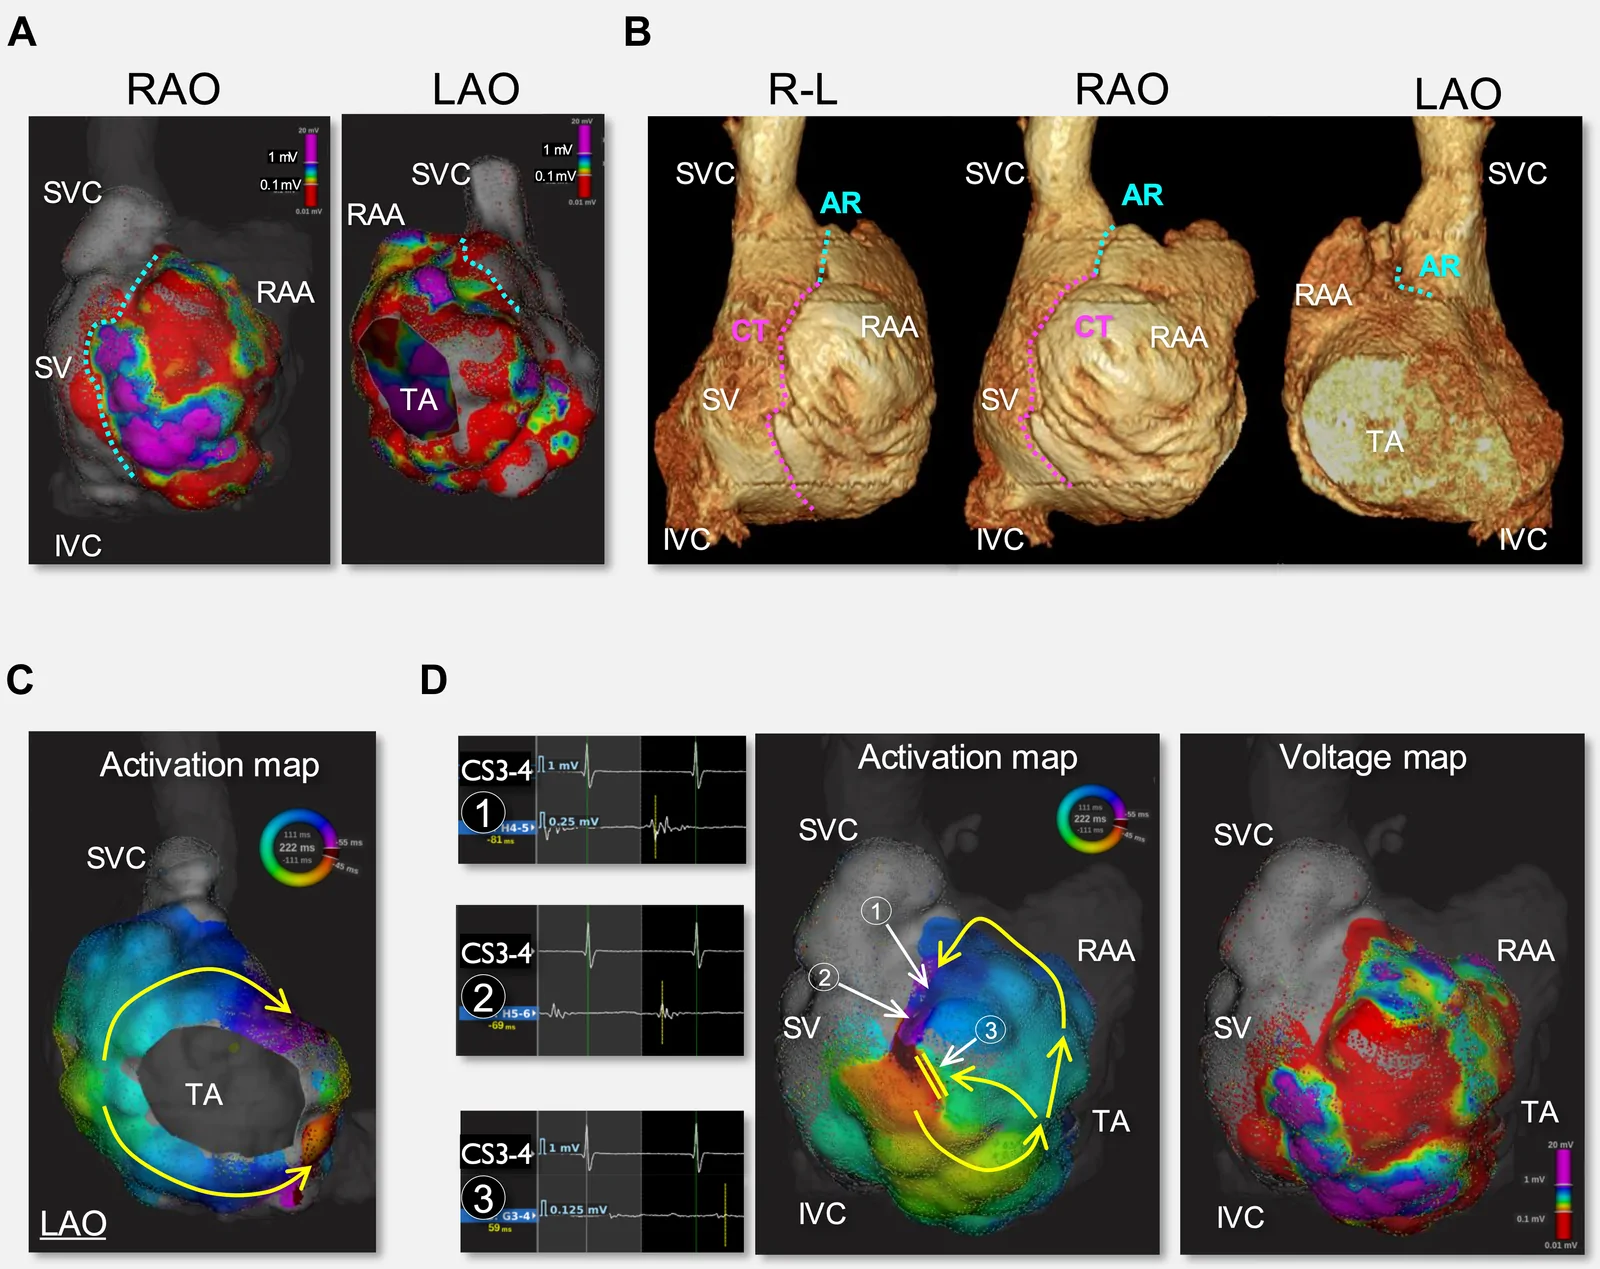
Electroanatomical Mapping and 3-Dimensional Computed Tomography Images (A) Voltage map of the right atrium during atrial tachycardia. Extensive low-voltage areas are observed in the sinus venarum (SV) and right atrial appendage (RAA). A confidence mask cutoff of 0.02 mV was applied during voltage mapping. The continuum of right atrial muscular ridge, encompassing an arcuate ridge (AR) (purple dashed lines) and the crista terminalis (CT) (cyan dashed lines), separates the SV and RAA. (B). Three-dimensional computed tomography images. The right atrial muscular ridge (cyan dashed lines) separates the SV and RAA. (C). Activation map viewed from the left anterior oblique (LAO). (D) Modified right anterior oblique (RAO). Fractionated electrograms are observed at sites corresponding to the AR (site 1) and the CT (site 2). Site 3 shows double potentials reflecting conduction block in the anteroposterior direction. Yellow arrows indicate the direction of wavefront propagation. HRA = high right atrium; IVC = inferior vena cava; R-L = right-to-left; SVC = superior vena cava; other abbreviations as in Figure 1.
